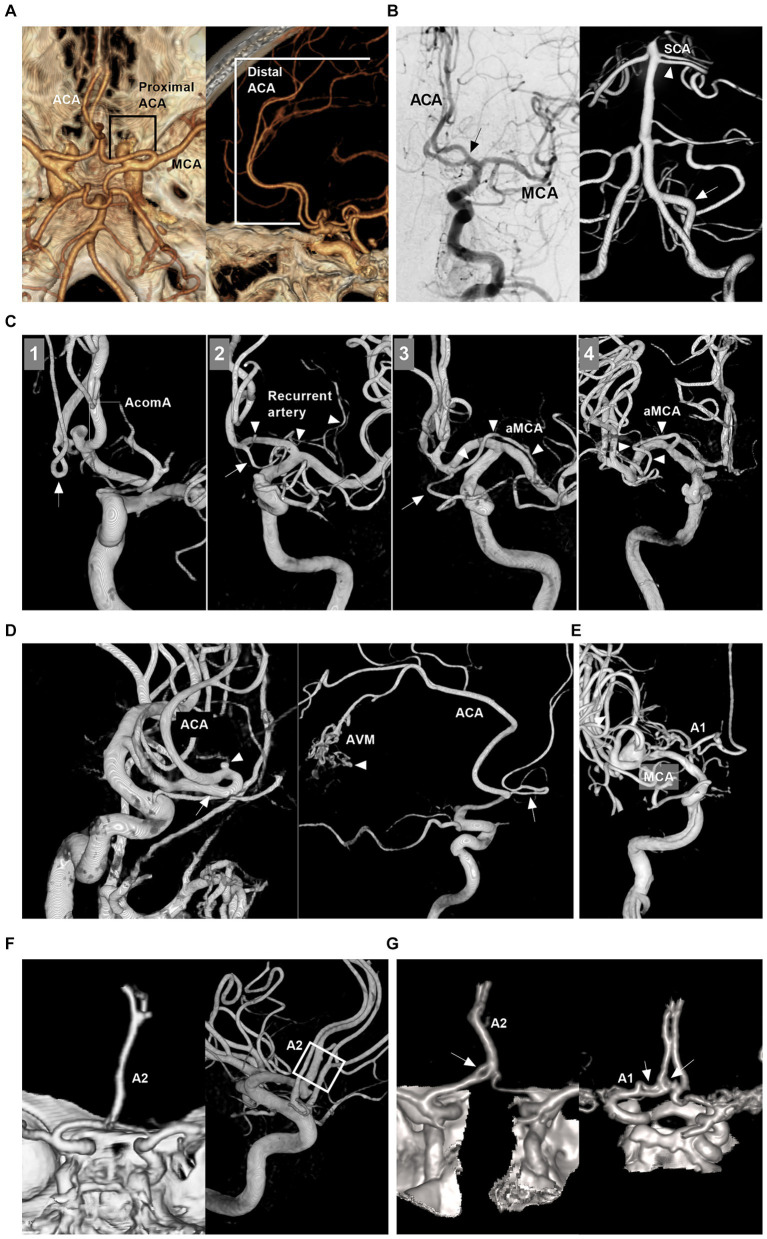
Intracranial ACA anatomy and anomalies. (A) CTA of superior to inferior (left panel) and lateral (right panel) views showing proximal and distal segments of the ACA. (B) Left panel: DSA of the ICA showing the early bifurcation (arrow) of the ACA at A1 segment; Right panel: 3D-DSA of the VA showing a large fenestration (arrow) of the VA and double SCAs (arrowhead); Images of left and right panels were from the same patient. (C) Panel 1: 3D-DSA of ICA showing the cortical branch from the AcomA; Panel 2: 3D-DSA of ICA showing that the recurrent artery of Heubner (arrowheads) and cortical artery (arrow) shared a common trunk from distal A1 segment; Panel 3: 3D-DSA of ICA showing that the aMCA (arrowheads) and cortical artery (arrow) shared a common trunk from distal the A1 segment. Panel 4: 3D-DSA of ICA showing that the aMCA (arrowheads) from the A1 segment near its origin. (D) Left panel: 3D-DSA of ICA showing proximal ACA course along the olfactory nerve (arrow) with an aneurysm (arrowhead) on it; Right panel: 3D-DSA of ICA showing the persistent olfactory artery (arrow) and an AVM (arrowhead). (E) 3D-DSA of ICA showing that the ACA originated from the lenticulostriate artery. (F) Left panel: CTA showing the azygos ACA (arrow); Right panel: 3D-DSA of ICA showing the triplication of ACA (frame). (G) Left panel: CTA showing a fenestration (arrow) of the A1 and the azygos ACA; Right panel: CTA showing two fenestrations (arrows) of A1 and AcomA. A1 and A2, first and second segments; ACA, anterior cerebral artery; AcomA, anterior communicating artery; aMCA, accessory middle cerebral artery; AVM, arteriovenous malformation; 3D, three-dimensional; CTA, computed tomography angiography; DSA, digital subtracted angiography; ICA, internal carotid artery; SCA, superior cerebellar artery; VA, vertebral artery.
